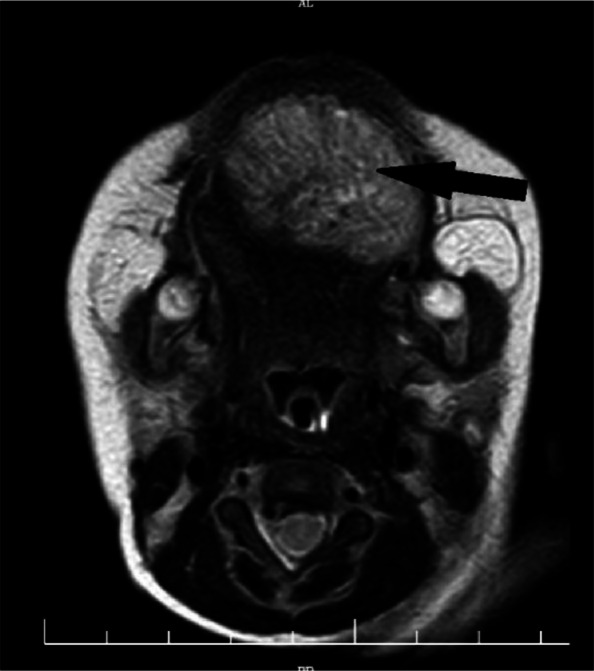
Axial T2-weighted MRI demonstrating a hyperintense lesion involving the anterior two-thirds of the tongue with internal flow voids (arrow), suggestive of a vascular malformation
